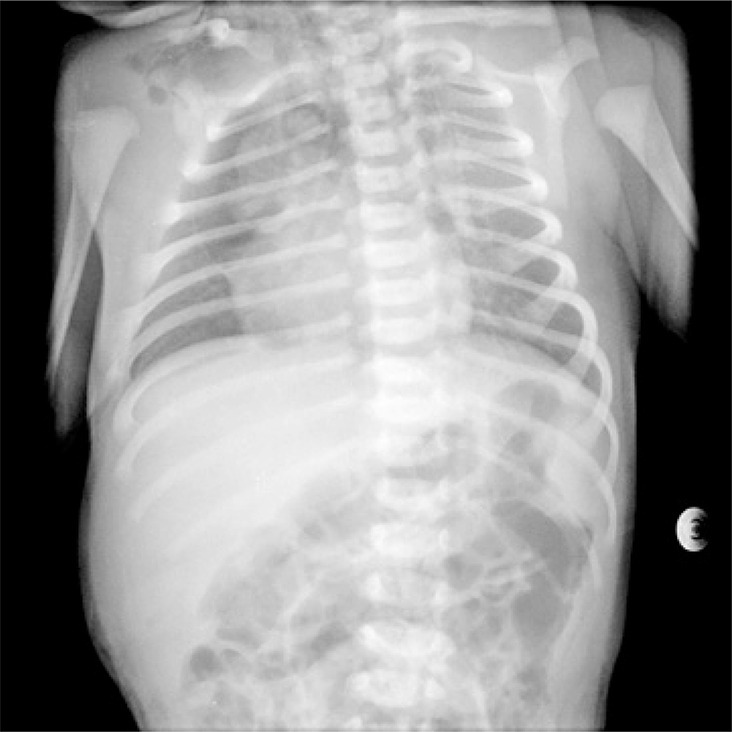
Chest radiography showing Spinnaker-Sail sign (wedge-shaped opacity extending to superior mediastinum, inferiorly delimited by a hyper-transparent area)
